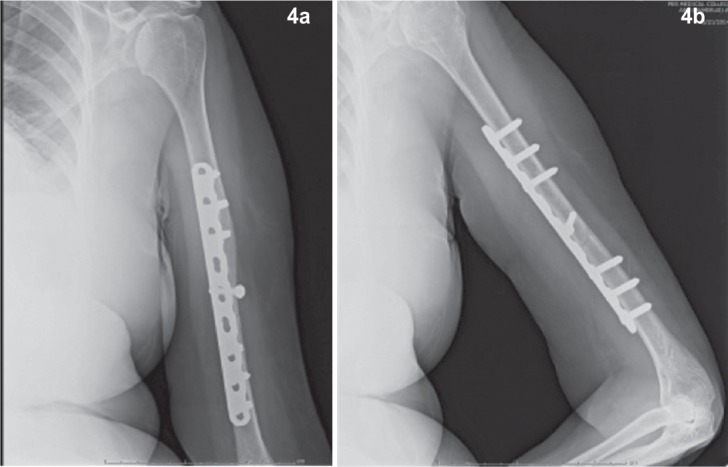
Anteroposterior and lateral radiographs at the end of 12 weeks of follow up:
